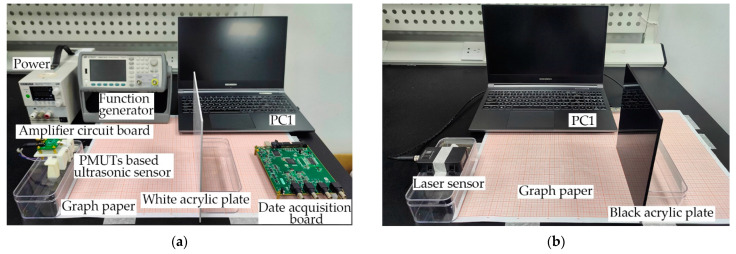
Static experimental setup of (a) the PMUTs-based ultrasonic sensor and (b) the laser sensor.
